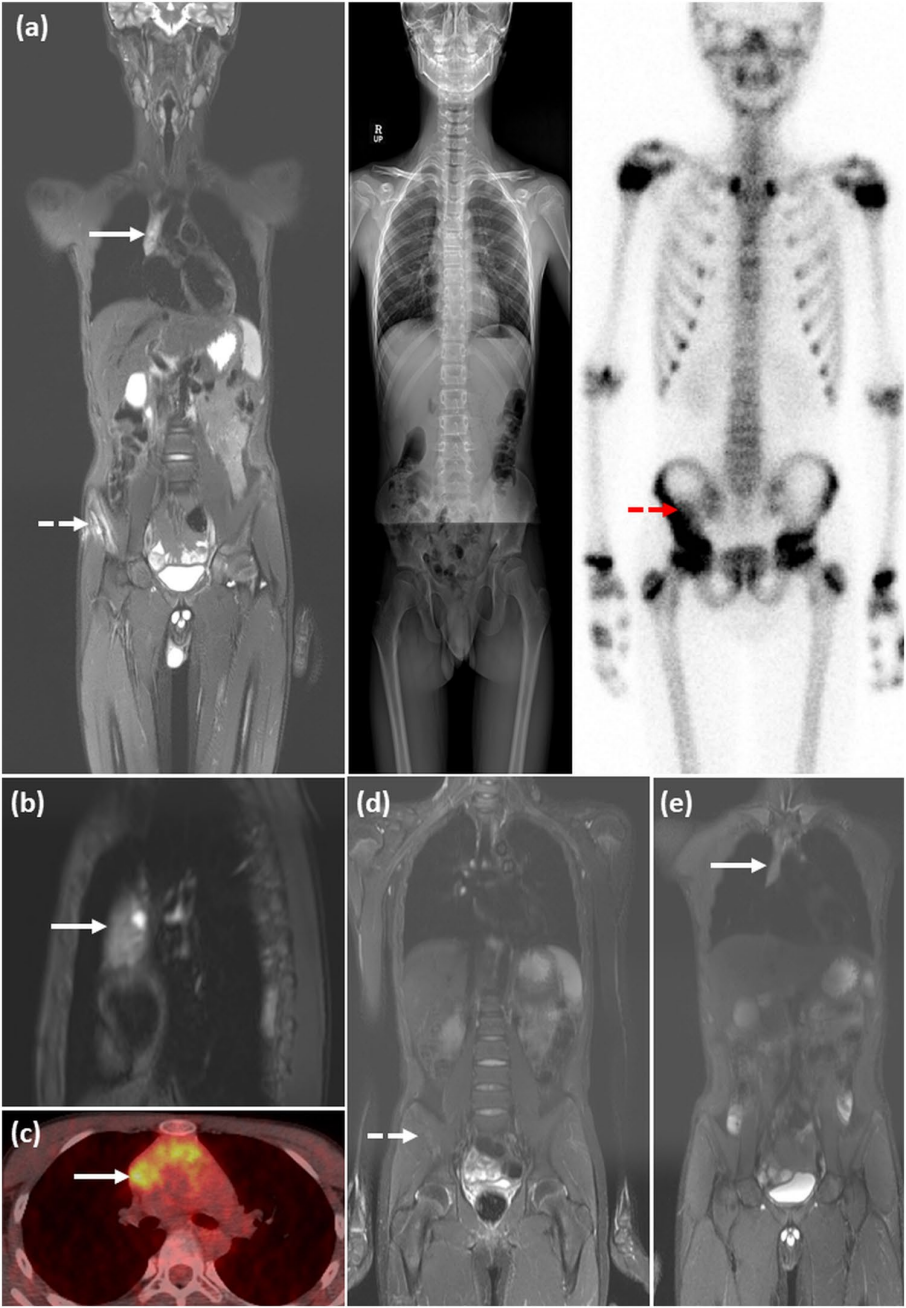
A representative case of an 11-year-old girl with a pathologically-confirmed LCH lesion in the right iliac bone. (a) Coronal STIR image from the WB-MRI (left), trunk and lower extremity images of skeletal survey (middle), and anterior view of bone scan (right), obtained for the initial evaluation of the extent of the disease, are shown. A known LCH lesion was detected on WB-MRI and bone scan (dashed arrows), but not on the skeletal survey, probably because of overlying bowel contents. WB-MRI also revealed heterogenous signal intensity with a tiny cystic portion in a slightly enlarged thymus (solid arrow), which was well-visualized on the sagittal STIR image (b), suggesting thymic involvement of LCH. However, thymic involvement could not be detected on the skeletal survey or bone scan. (c) PET-CT scan showing heterogenous increased FDG uptake in the thymus (arrow), raising the possibility of thymic involvement of LCH. (d,e) Follow-up WB-MRI after 6 months of treatment showing decreased size of the lesion in the right iliac bone (dashed arrow, d), and the near-normalized size and signal intensity of the thymus (arrow, e). On the risk stratifications, the patient was under-classified as single system LCH without multiple bone lesions or CNS risk lesions on the skeletal survey and bone scan, but correctly classified as multisystem LCH without risk organ involvement on WB-MRI.
